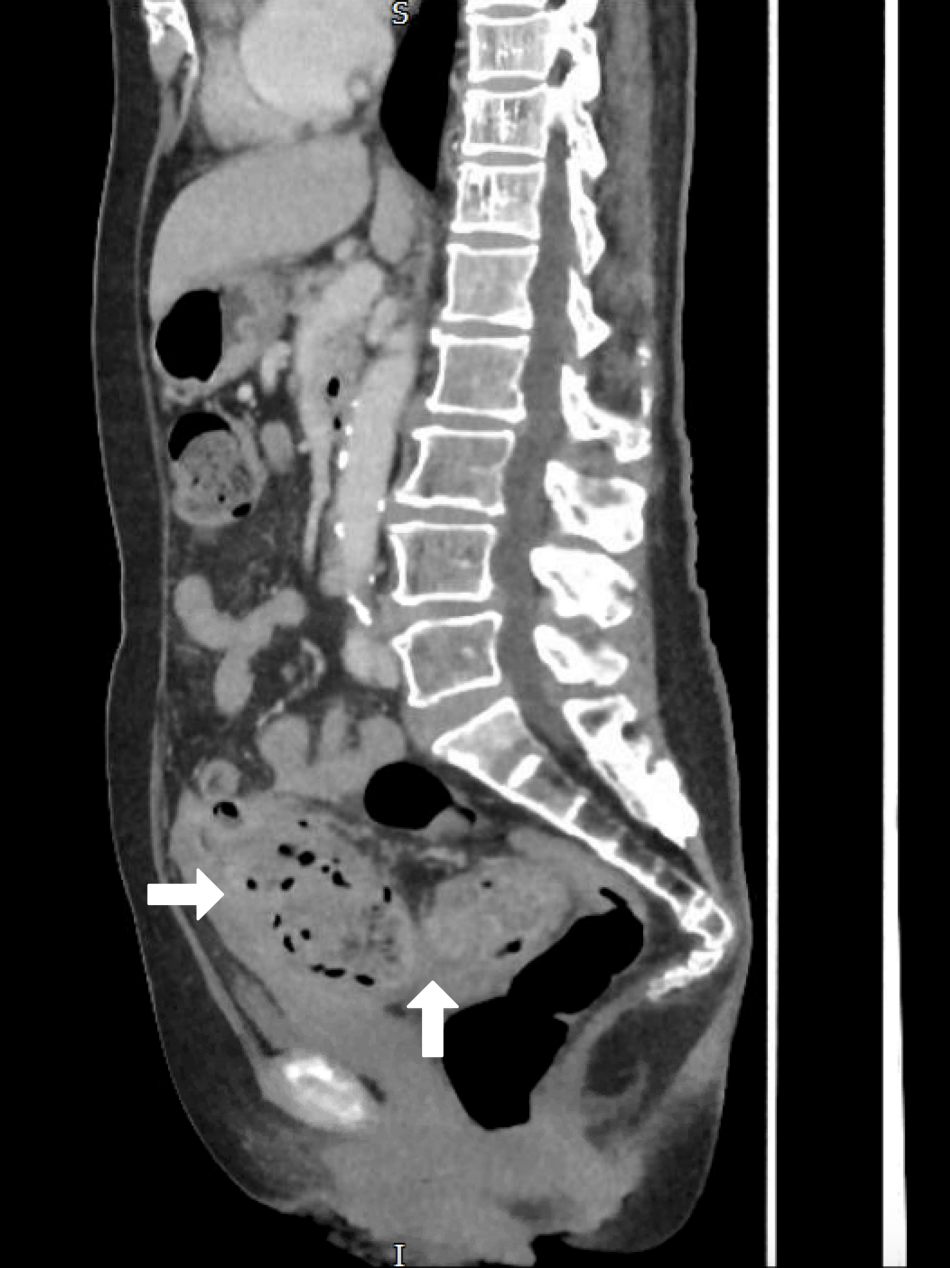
CT Abdomen & Pelvis with Contrast (Saggital View)
